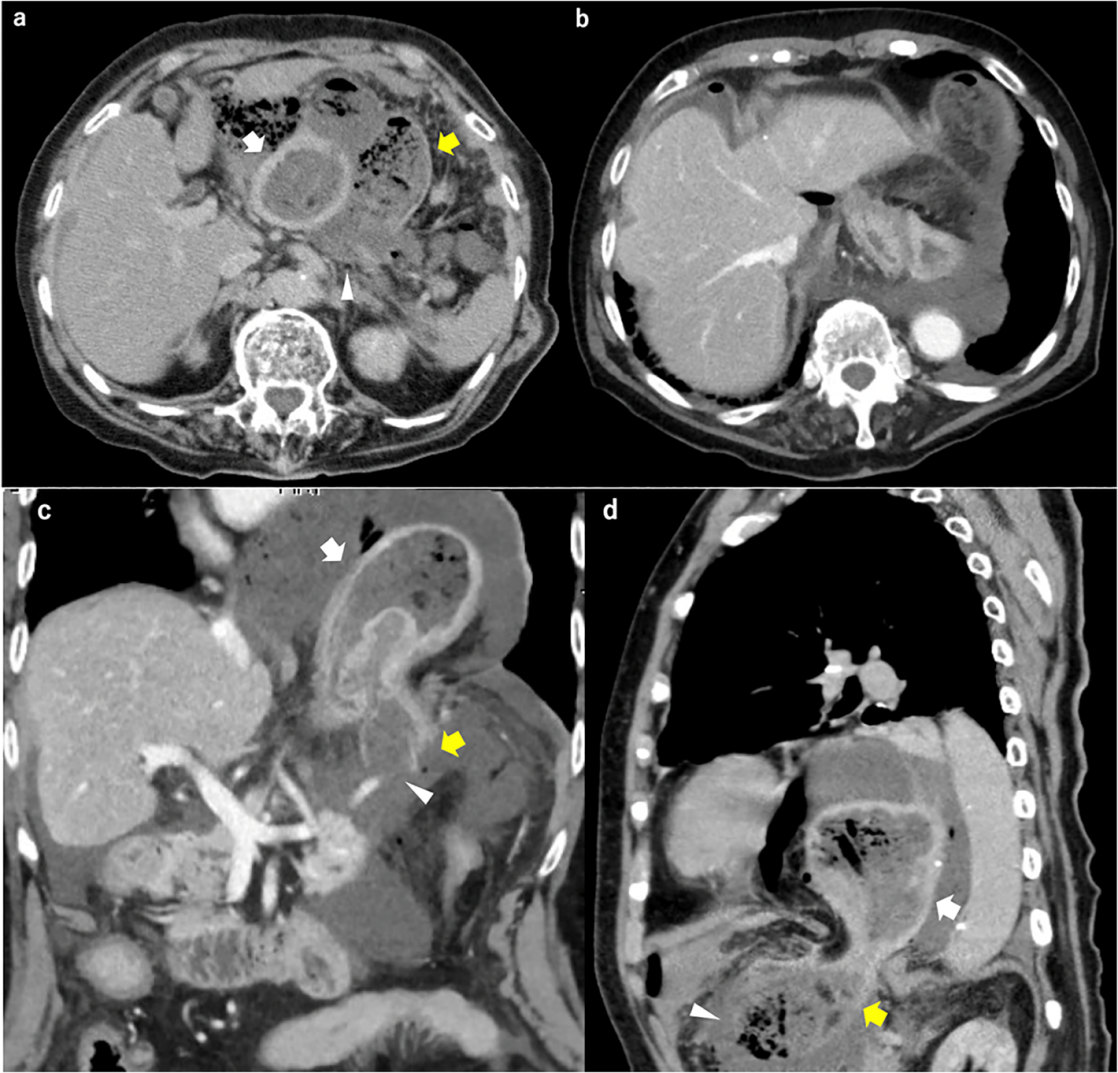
Preoperative computed tomography scan. Preoperative computed tomography scan (a, c, d) showing the gastric fundus and upper body incarcerated within the hiatal hernia (white arrow). The perforation (arrowhead) was located at the fundus outside the sac, which was locally ischemic and necrotic owing to complete cessation of blood supply (yellow arrow). All arteries, including the short gastric artery, were not clear on computed tomography, but arteries flowed into the sac at the hernia orifice (b, c, d) and disappeared
